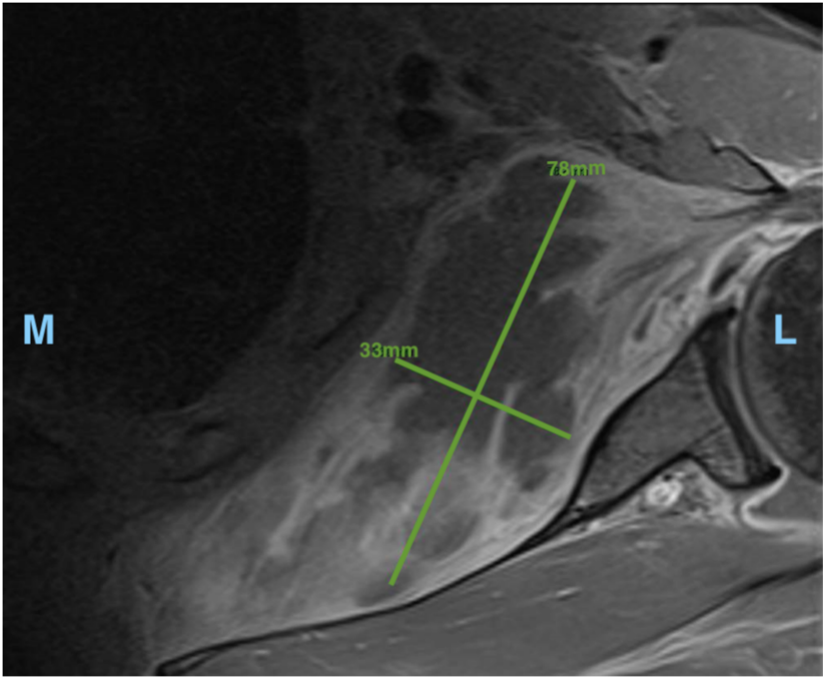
Transverse magnetic resonance imaging (MRI) of left shoulder. Measurements of largest locule (78 × 33 mm). L, lateral. M, medial.
